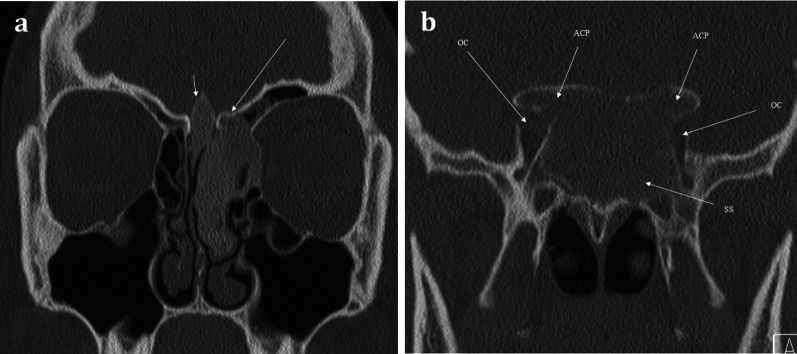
Fibrous Dysplasia. 24-year-old female patient with history of diabetes and café au lait spots presenting with long standing anosmia and nasal congestion, and acute left sided visual loss. Coronal CT images showing expansile multifocal osseous lesions with typical internal ground-glass matrix, involving the left cribriform plate (long arrow, a) and crista galli (short arrow, a). This is causing remodelling of the olfactory fossae and is affecting the olfactory pathway. There are similar expansile lesions affecting the anterior clinoid processes (ACP) and the sphenoid sinus (SS) which causes compromise of both optic nerve canals (OC), worse on the left—this accounts for the visual loss in the left eye. The patient was diagnosed with McCune–Albright syndrome (polyostotic fibrous dysplasia with cutaneous and endocrine abnormalities), and surgical decompression of the left optic canal was performed
